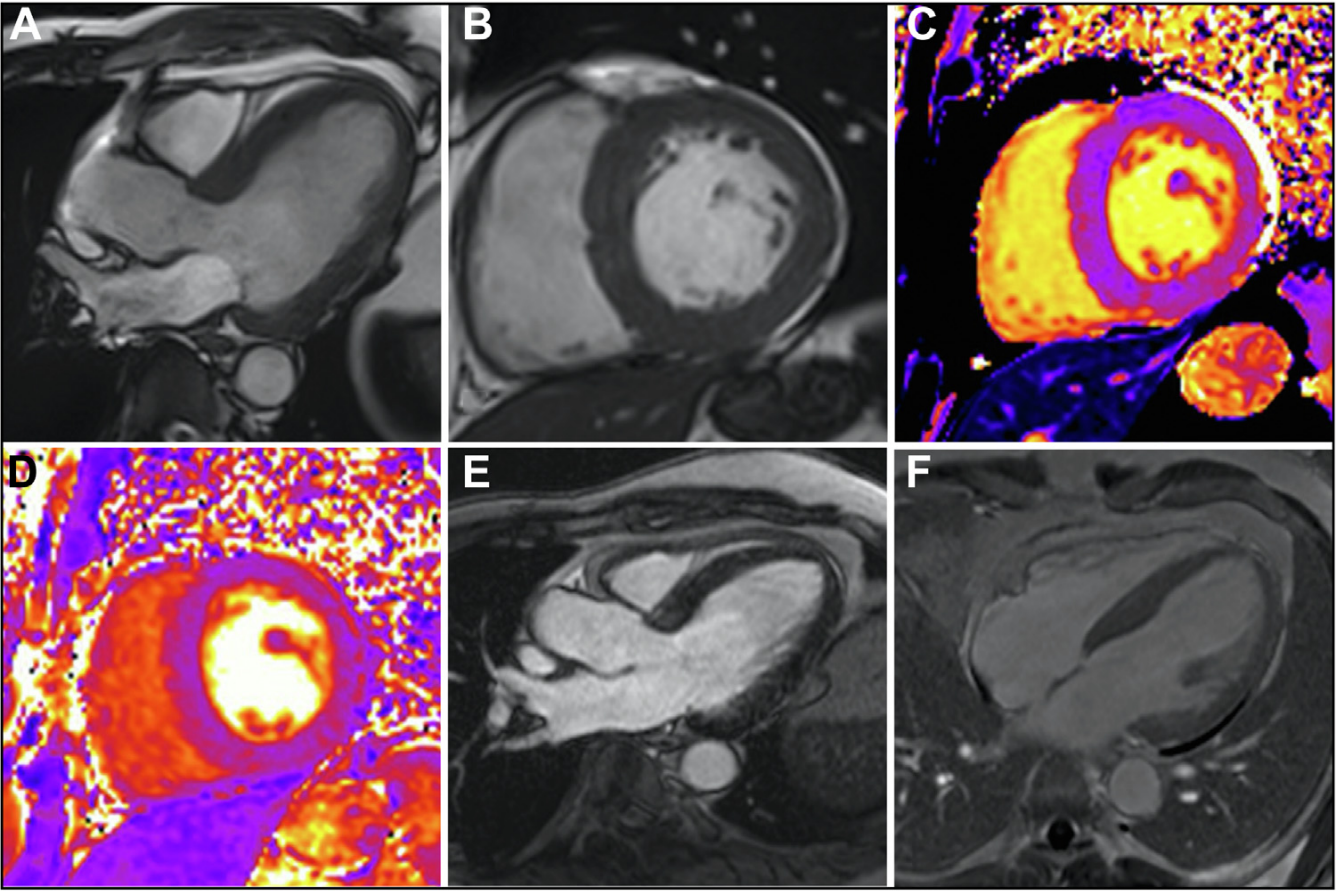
Case #2 Cardiac Magnetic Resonance Images (A) Three-chamber and (B) basal short-axis balanced steady-state free precession images. (C) Basal short-axis T_1_ map (modified look-locker inversion recovery). (D) Basal short-axis T_2_ map. (E) Three-chamber phase-sensitive inversion recovery late gadolinium enhancement image acquired in the late phase following gadolinium administration. (F) Basal short-axis phase-sensitive inversion recovery late gadolinium enhancement image. Images are from a cardiac magnetic resonance scan conducted before the hybridized imaging.
